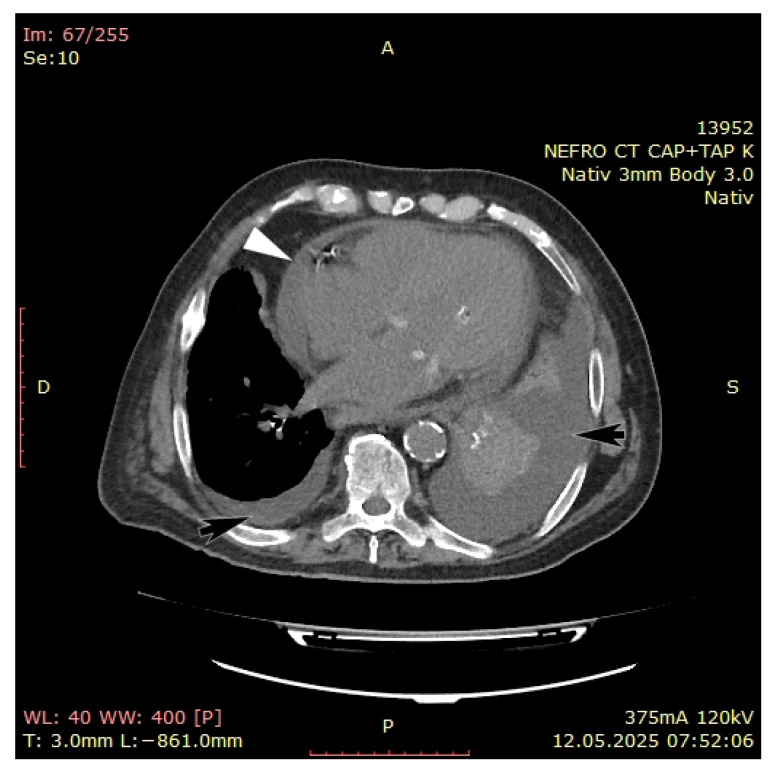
Follow-up CT scan with contrast agent highlighted an accumulation of fluid of 18 mm adjacent to the right atrium (white arrow); pleurisy of 14 mm on the right side and 3 mm on the left side (black arrows).
